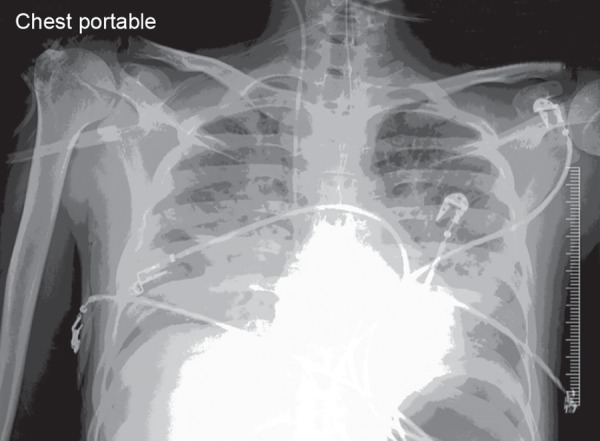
X-ray chest of patient on day 2 of intensive care unit (ICU) admission when he developed pulmonary edema.
